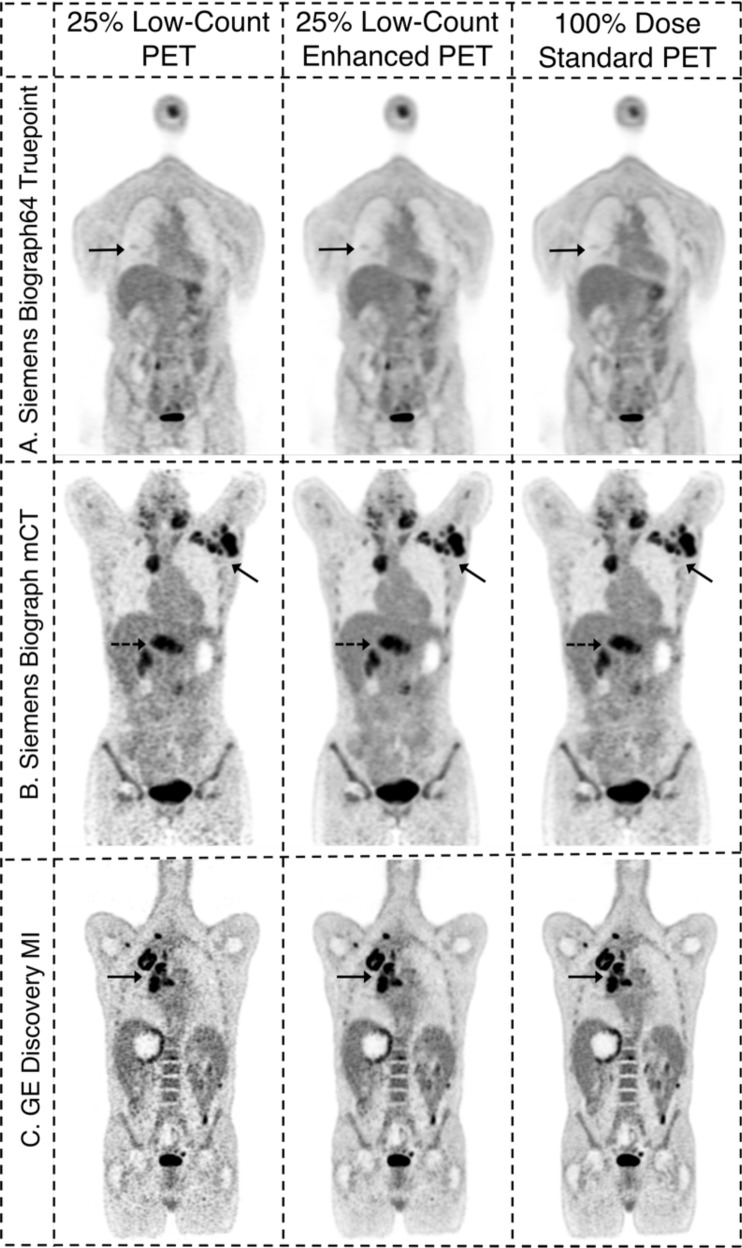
Example 25% low-count PET images, the 25% low-count-enhanced images, and the corresponding standard images for all three scanners for subjects with body mass index (BMI) under 30. A A 66-year old male with BMI of 27 scanned on a Siemens Biograph64 Truepoint for oropharyngeal cancer (solid arrow pointing to a distant lung metastasis). B A 34-year-old female with BMI of 20 scanned on a Siemens Biograph mCT for lymphoma (solid arrow pointing to metastatic lymph nodes and dashed arrow pointing to lesion in the spleen). C A 58-year-old male with BMI of 23 scanned on a GE Discovery MI with lung cancer (solid arrow). For all subjects, all low-count images appear considerably noisier compared with the low-count-enhanced and standard images. Note: SUV display scale is 0–7.
